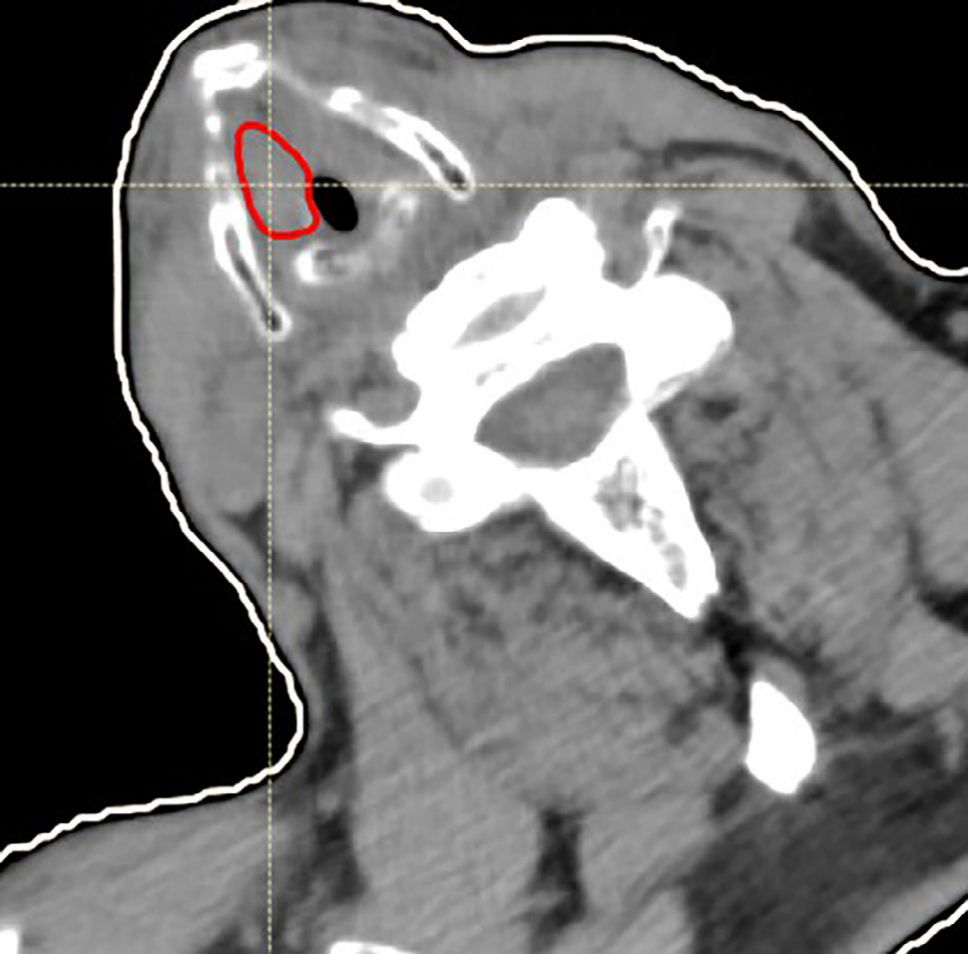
Axial CT image of the patient treated with BNCT. The GTV location is outlined in red.
